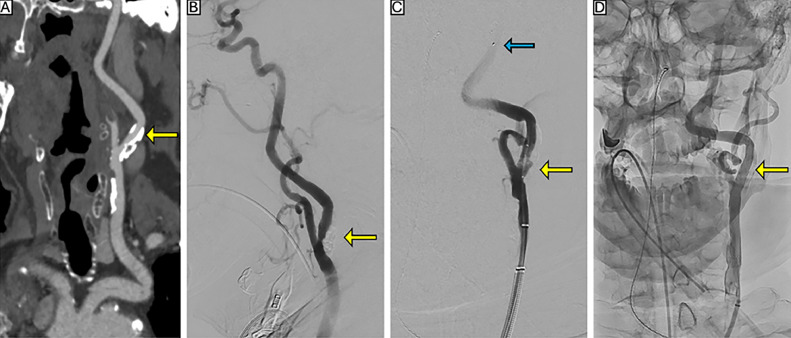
(A) CTA reconstruction demonstrating a heavily calcified plaque at the origin of the left internal carotid artery (ICA), causing approximately 80% stenosis by NASCET criteria (yellow arrow). (B) Digital subtraction angiography confirming the location and severity of the calcified lesion (yellow arrow). (C) Intravascular lithotripsy performed using a 4 mm × 12 mm ShockWave C2+ balloon (yellow arrow) with the distal embolic protection device positioned beyond the lesion in the ICA (blue arrow) and (D) Completion angiography following deployment of an 8 mm x 29 mm stent, demonstrating successful luminal restoration (yellow arrow).
